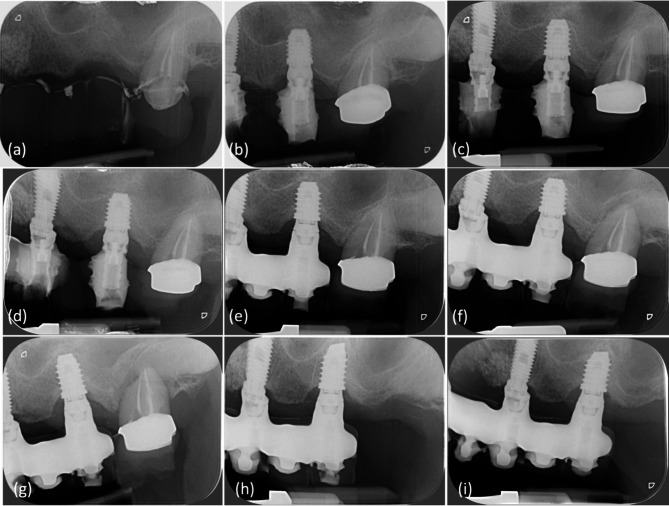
A case of full-arch immediate loading including a 5 mm diameter and 6 mm length implant placed in position 25: (a) preoperative periapical radiograph, (b) periapical radiograph taken at the time of placement and immediate loading (a 4.1 mm diameter and 3 mm height intermediate abutment was placed at implant surgery); (c) one-year follow-up radiograph; (d) two-year follow-up radiograph; (e) 3-year follow-up radiograph (the final restoration is in place); (f)-(i) periapical radiographs at 4–7 years of follow-up
